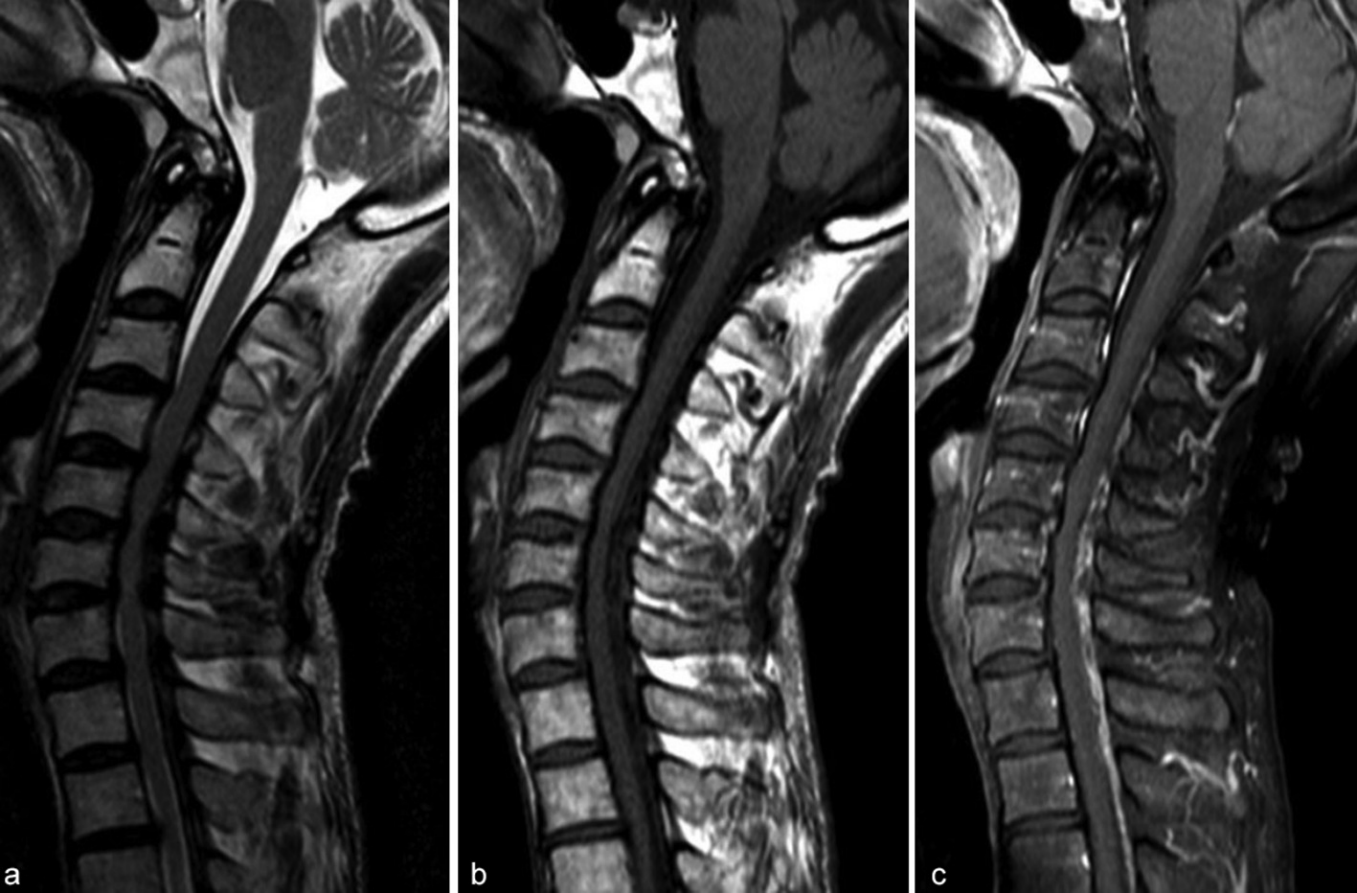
Preoperative MR imaging of the cervical spine. The lesion shows hypointensity on both (a) *T*_2_ and (b) *T*_1 _weighted images which causes spinal canal stenosis. (c) Sagittal post-contrast *T*_1_ weighted imaging demonstrates heterogeneous enhancement.
